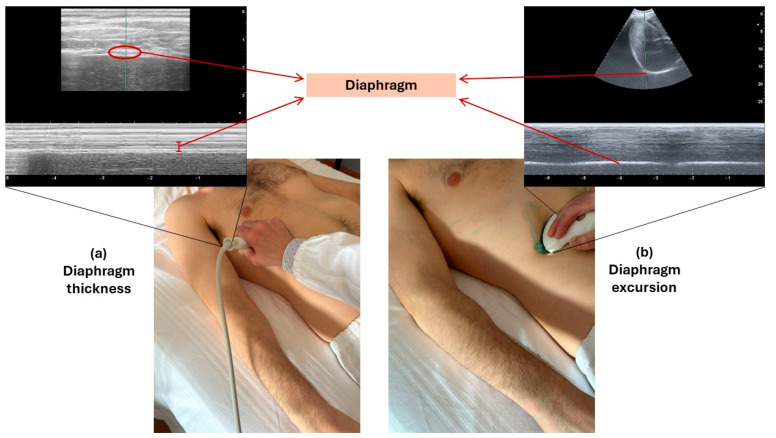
Ultrasound scans required for measuring diaphragm thickness (a) and diaphragm excursion (b). The linear ultrasound probe should be first put in the right eighth, ninth or tenth intercostal space, with an oblique or transversal scan, in order to visualize the zone of apposition as the area where the curtain sign appears under quiet inspiration. The diaphragm can be visualized as the structure between two parallel hyperechoic lines just below the curtain sign (a). Then, the convex probe should be put in the right subcostal region, directing the probe upwards in order to exploit the acoustic window of the liver. The diaphragm appears as the cupola-like hyperechoic structure surrounding the right liver lobe, exhibiting movements synchronous with respiration (b). Both diaphragm thickness and excursion need to be measured with the M-mode function of the ultrasound turned on, asking the patient to perform quiet breathing and maximal voluntary inspirations.
